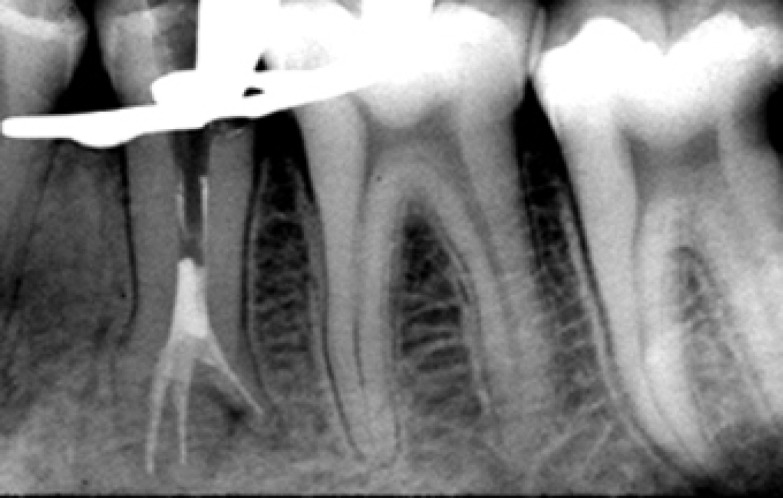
immediate postobturation radiograph
